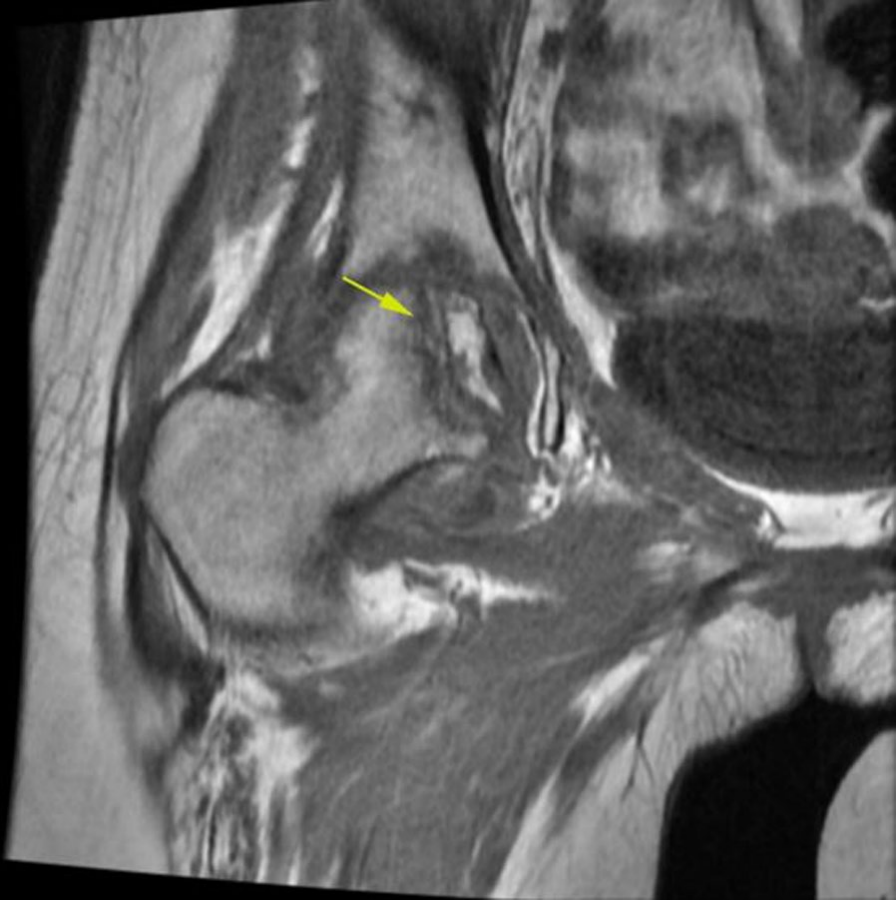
Coronal T1 non-fat sat MRI shows low signal serpiginous lines of femoral head compatible with femoral head AVN (yellow arrow).
